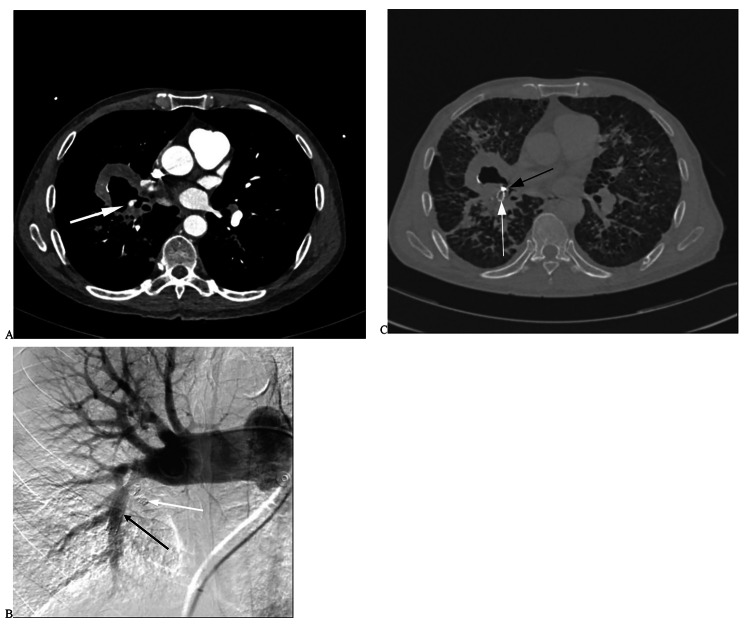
A patient with old tuberculosis was hospitalized with sudden massive hemoptysis for 1 day, and the volume of one-time hemoptysis was about 500ml. A. Chest enhanced CT shows cavity formation in the lower lobe of the right lung and PAP formation in pulmonalis lobar arteria (white arrow). B. No PAP was found after the stent (black arrow) assisted PAPE with a micros coils (white arrow) for the right lower pulmonalis lobar arteria. C. Postoperative chest CT scan shows the position of the stent (white arrow) and coils (black arrow)
